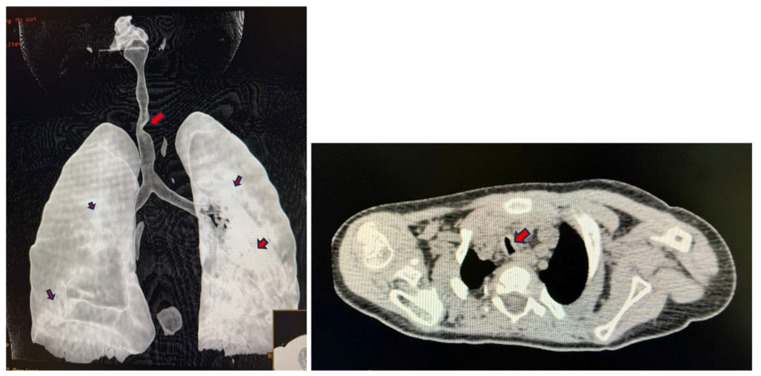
CT scan of chest and 3-dimensional reconstruction shows tortuous and narrowed trachea and patchy infiltrates marked by red arrows.
